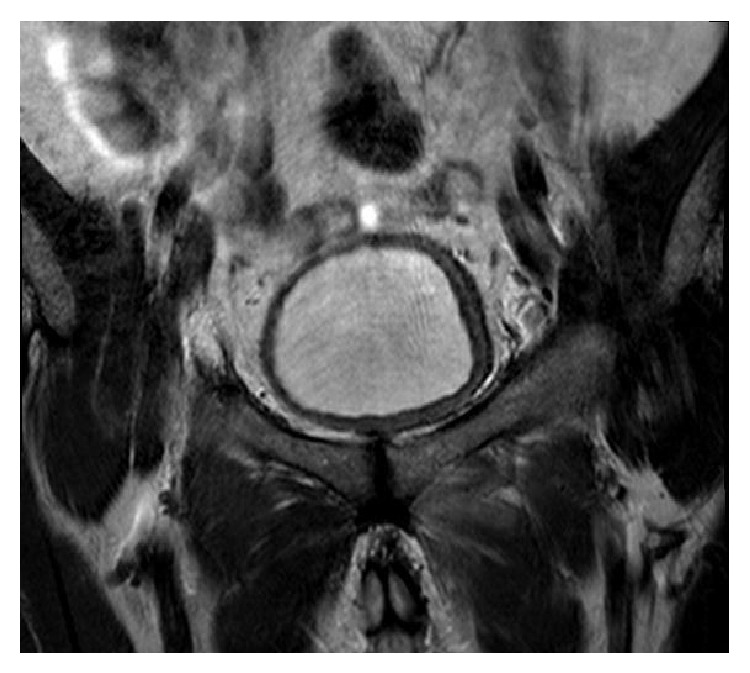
Pelvic MRI two months after completion of antibiotic therapy reveals no inflammatory change.
